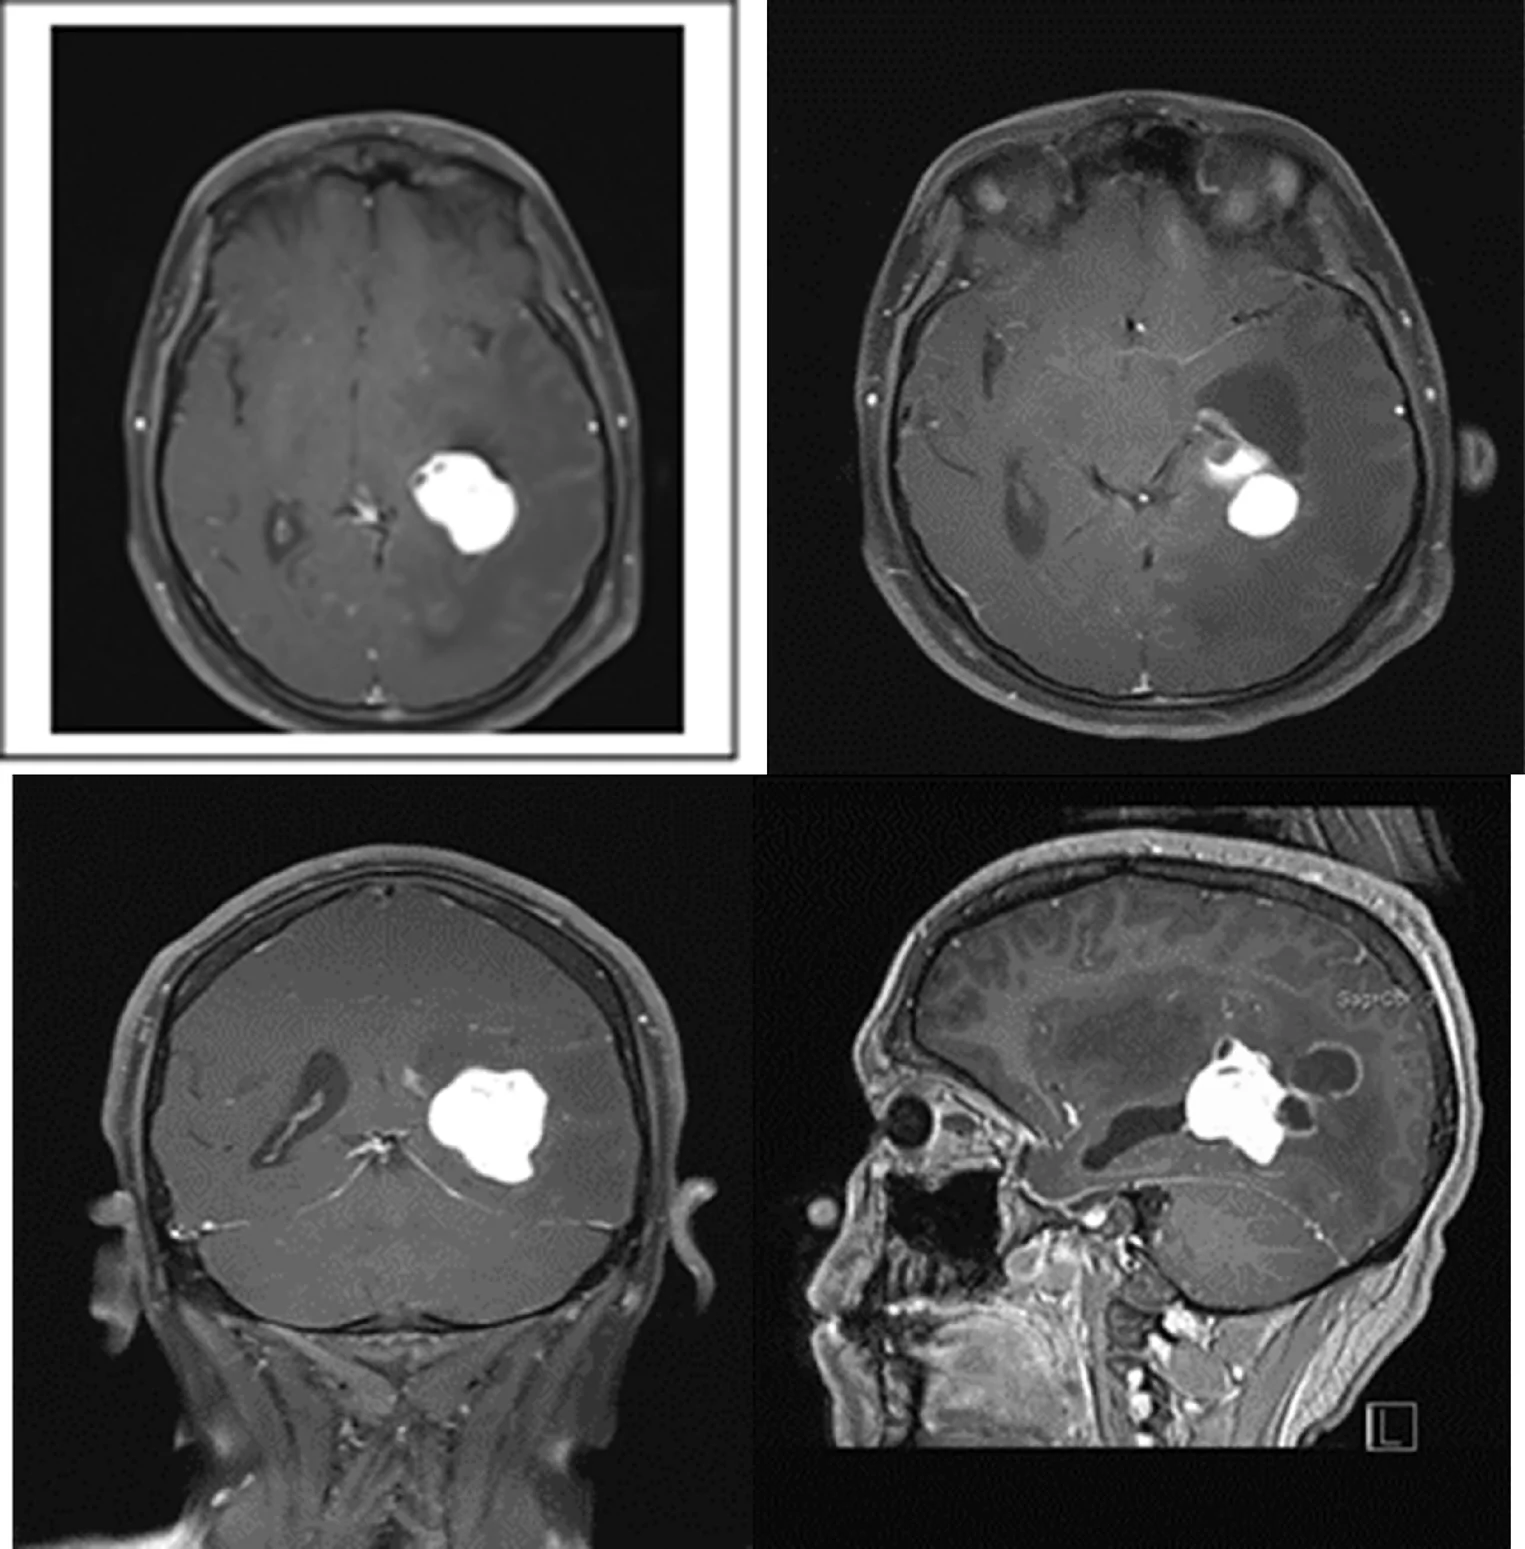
Preoperative: MRI axial, coronal, and sagittal with gadolinium contrast demonstrating left trigon of temporal horn intraventricular enhancing lobulated solid with cystic mass causing entrapment with hydrocephalous due to disconnection of temporal horn from the rest of ventricular system.
